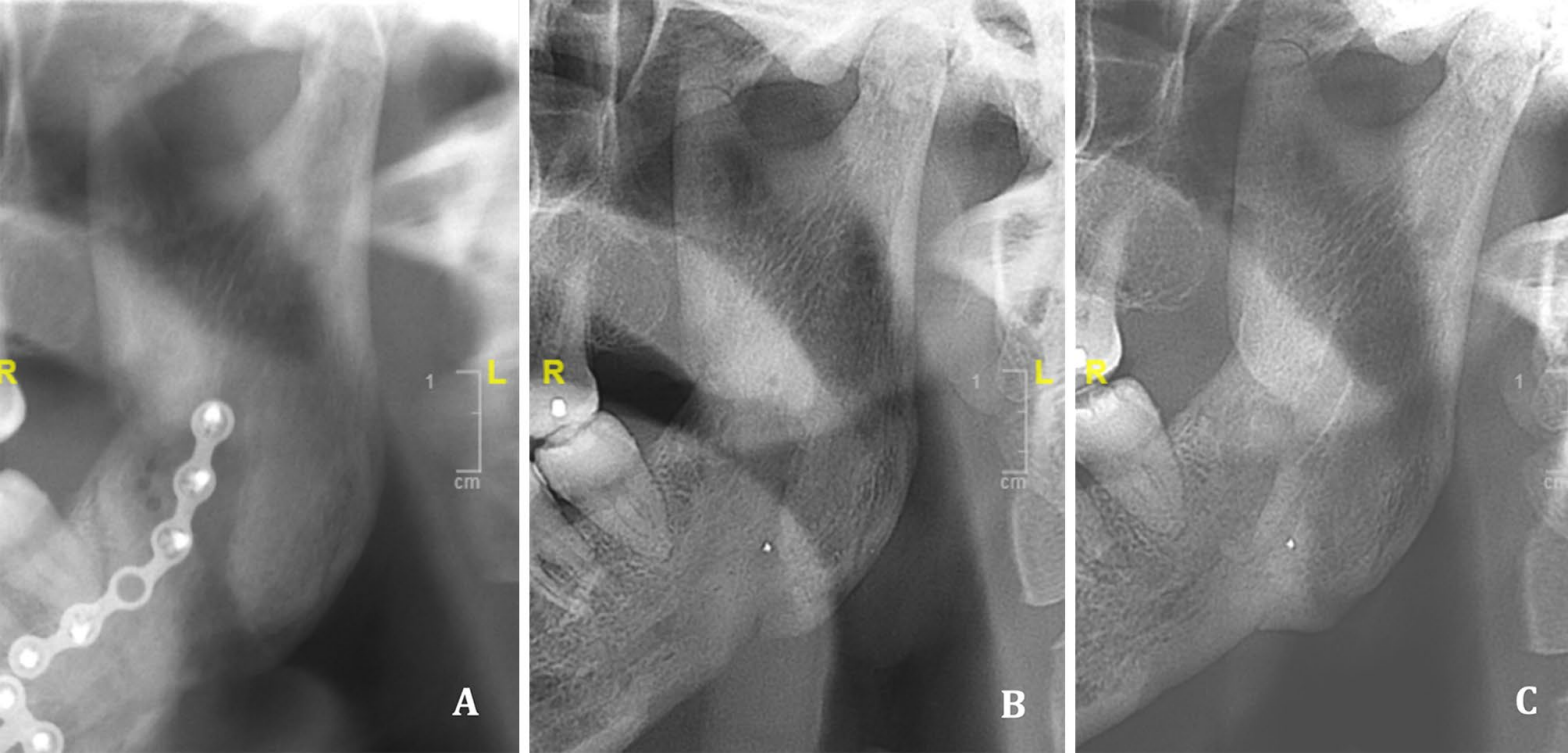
Radiographic evolution of osteomyelitis: diagnosis (A), 1 day after hardware removal and surgical curettage (B), and 1 year after the antibiotic treatment (C).
